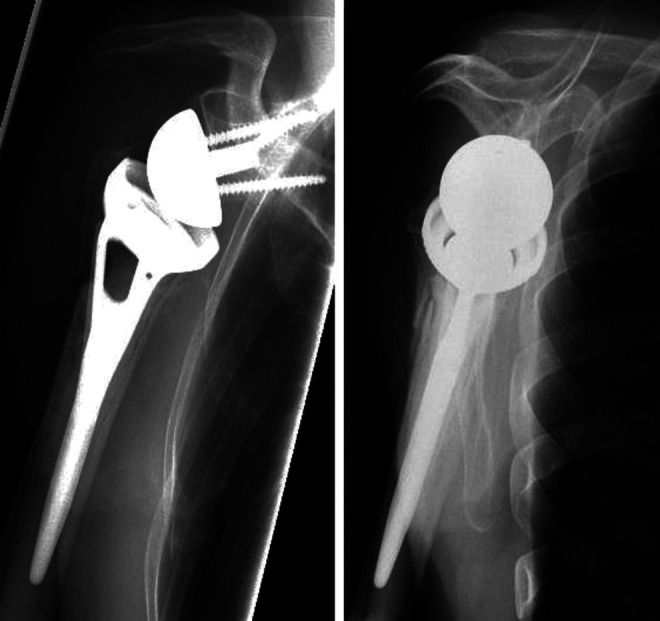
Complete integration of the tuberosities in RSA in a.p. and y-view radiographic view.
